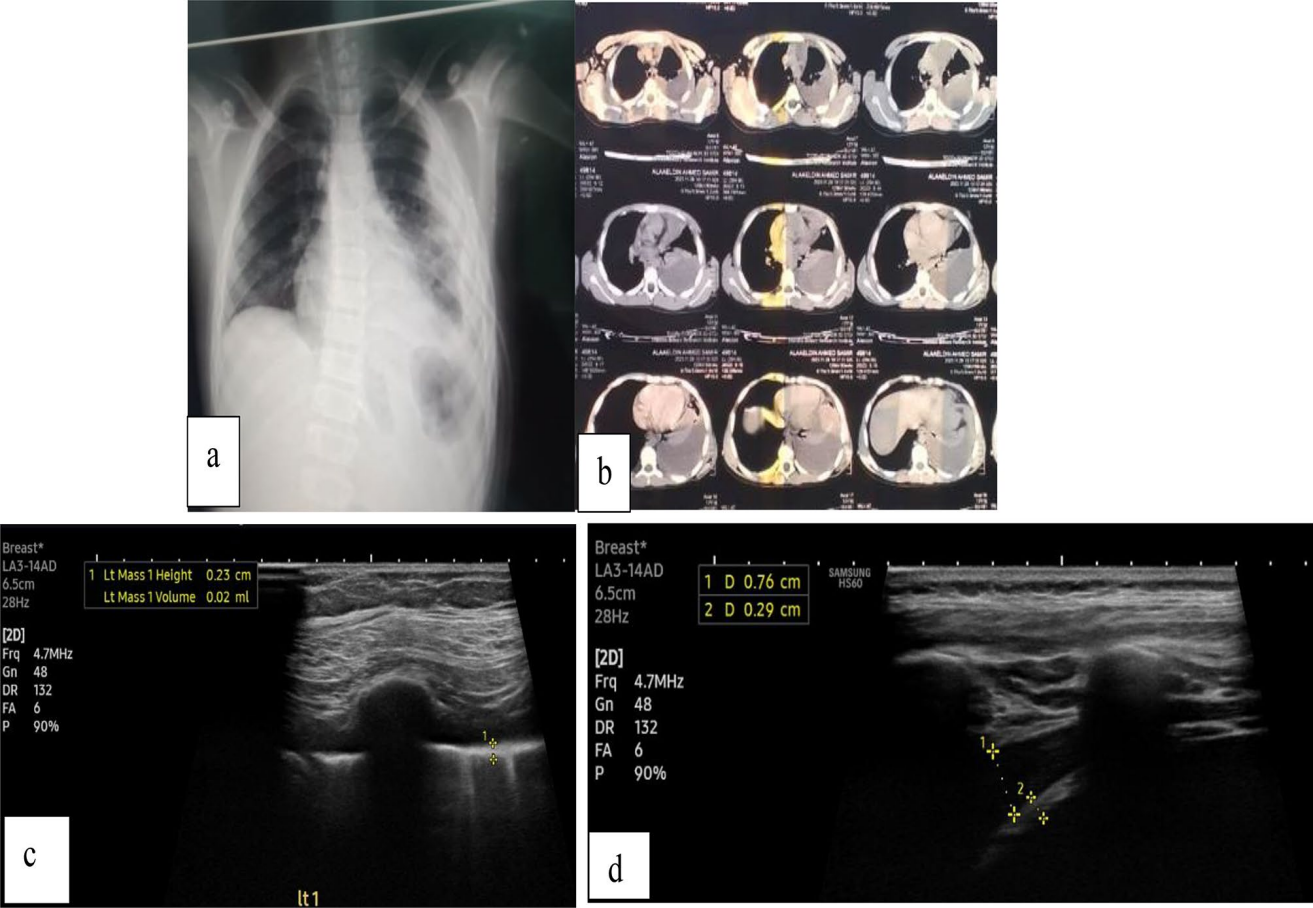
9 years old male boy presented by shortness of breath and diagnosed as CAP .(a) Plain chest x-ray PA view showing obliterated left costophrenic angle by density rising towards the axilla and left lateral density.(b) Non contrast CT chest done on admission showing moderate left sided pleural effusion with left lower lobar lung collapse.(c, d) Follow up lung U/S showing thickened pleura of the left lung, measuring 2.3–2.9 mm in thickness with still noted mild left sided pleural effusion
